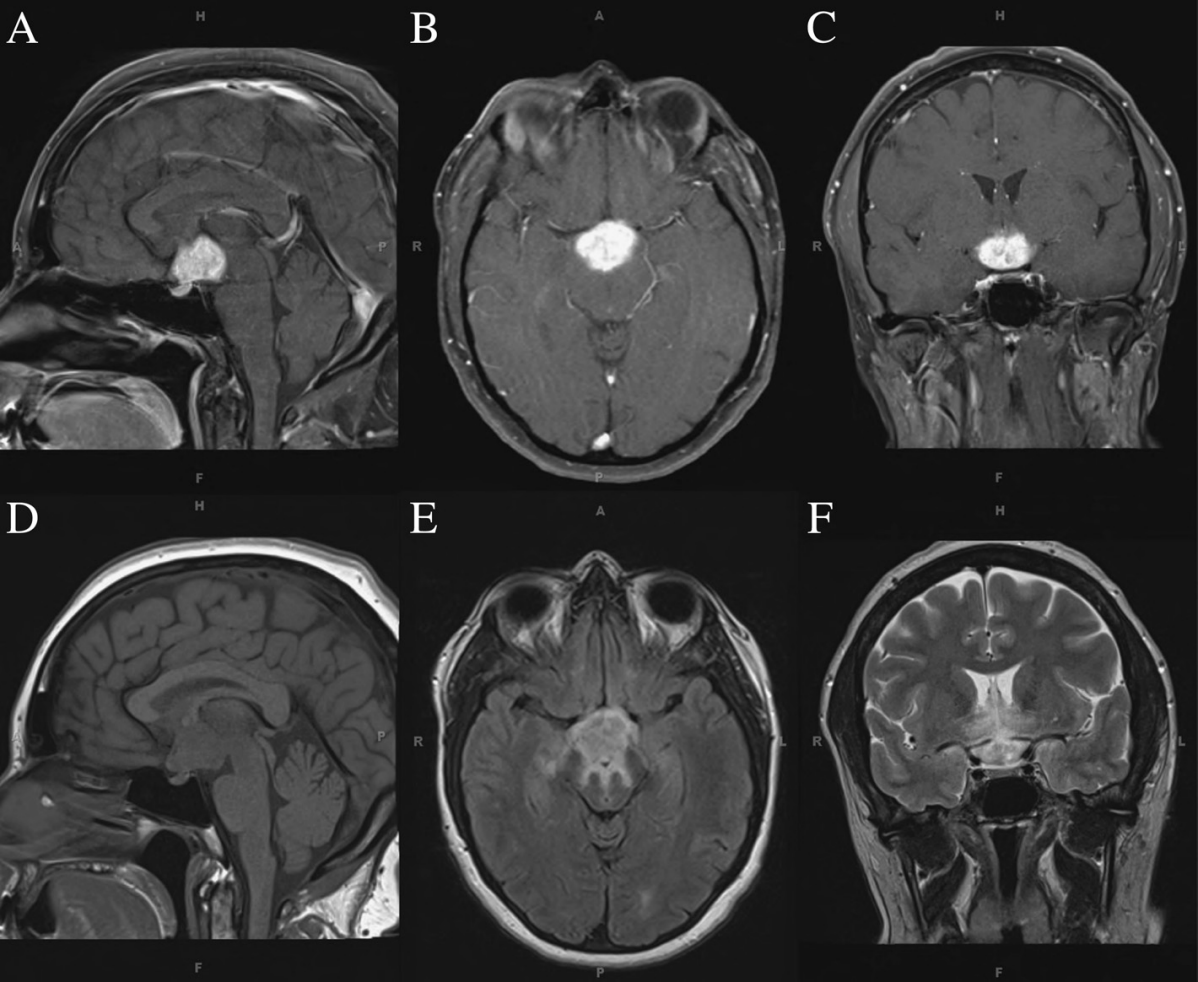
Radiographic imaging at the time of initial diagnosis. T1-weighted magnetic resonance image with (A, B, and C) and without (D) contrast demonstrate the large enhancing Langerhans cell histiocytosis lesion in the suprasellar region. Fluid attenuated inversion recovery (E) and T2-weighted imaging (F) demonstrate mild edema in the surrounding deep thalamic nuclei and brainstem.
